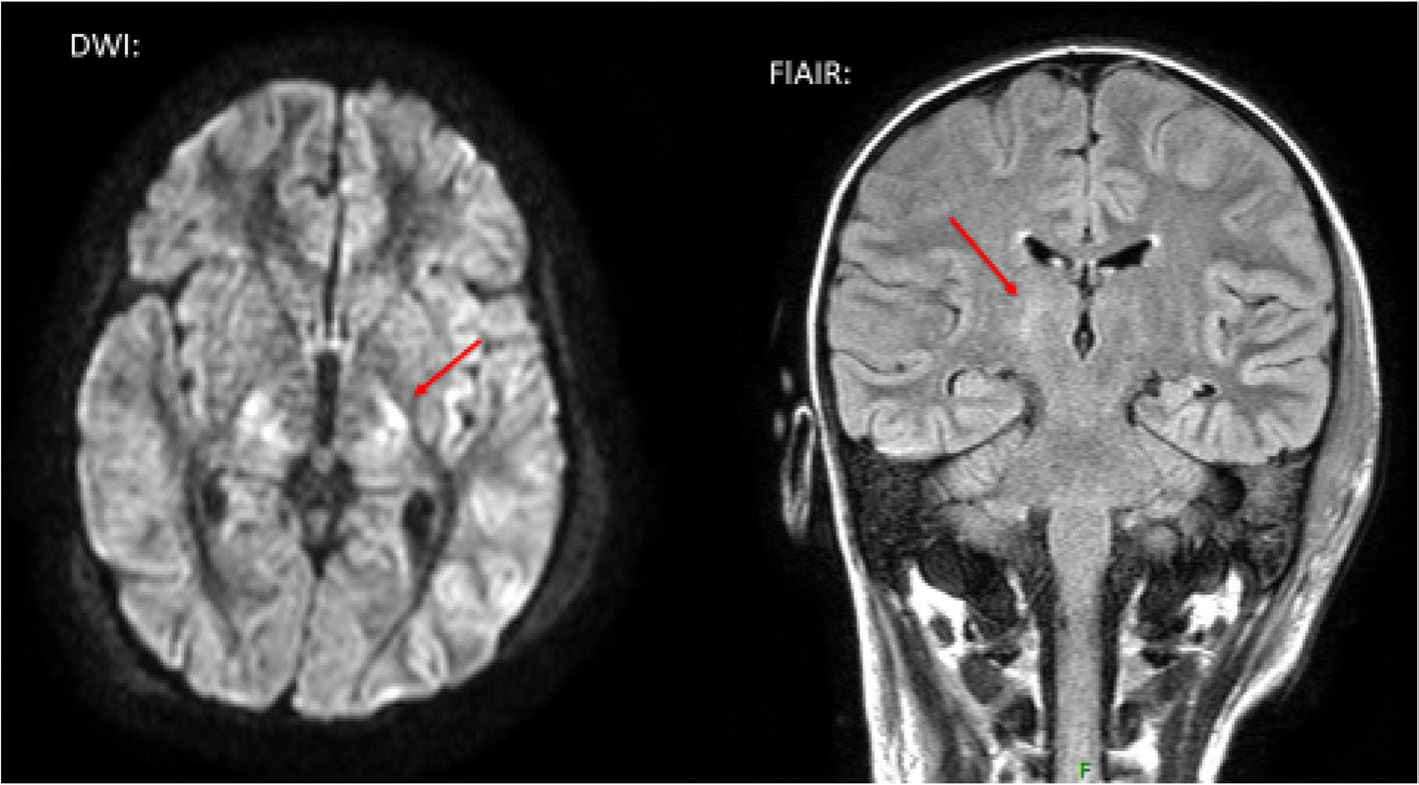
MRI of patient 3. On the MRI (FLAIR and DWI) images, a symmetric, hyperintense, banded abnormality can be seen in the area of ​​both thalamus, on the basis of which the abnormality may correspond to Percheron arteriopathy (arrow). The Percheron artery is a rare anatomical variant, in which both lateral vessels originate from the same main trunk, and its injury (vasculitis, thromboembolism) causes a symmetrical deviation. The symptoms, such as fluctuating cognitive impairment, aphasia, memory impairment, and right-sided motor symptoms were all detectable in our 3rd patient and corresponded to the abnormality seen on the MR image
